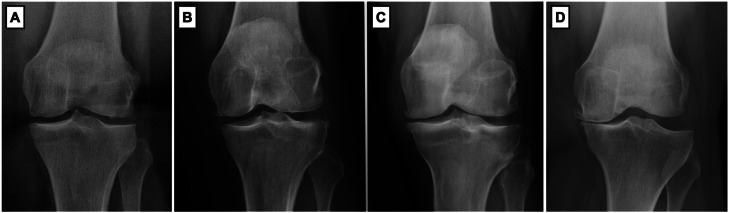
Knee radiographs classified by Kellgren-Lawrence system. (A) Kellgren-Lawrence 1 (doubtful joint space narrowing and possible osteophytic lipping); (B) Kellgren-Lawrence 2 (definite osteophytes and possible joint space narrowing); (C) Kellgren-Lawrence 3 (moderate multiple osteophytes, definite narrowing of joint space, some sclerosis and possible deformity of bone ends); (D) Kellgren-Lawrence 4 (large osteophytes, marked narrowing of joint space, severe sclerosis, and definite deformity of bone ends).
